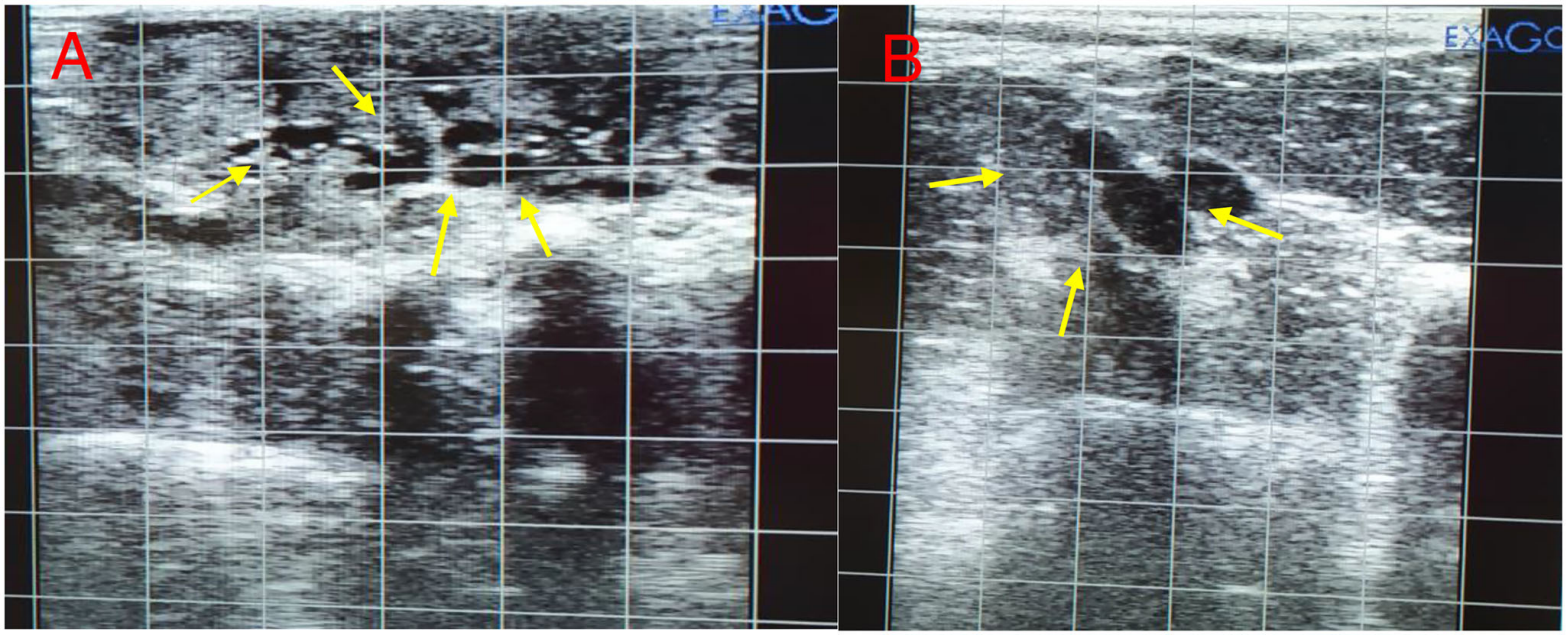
Ultrasound images on Day 15 of the experiment (72 h after synchronization) show the number of follicles in Ghezel ewes. (A) Yellow arrows show the developing follicles. (B) Yellow arrows show the preovulatory follicles.
